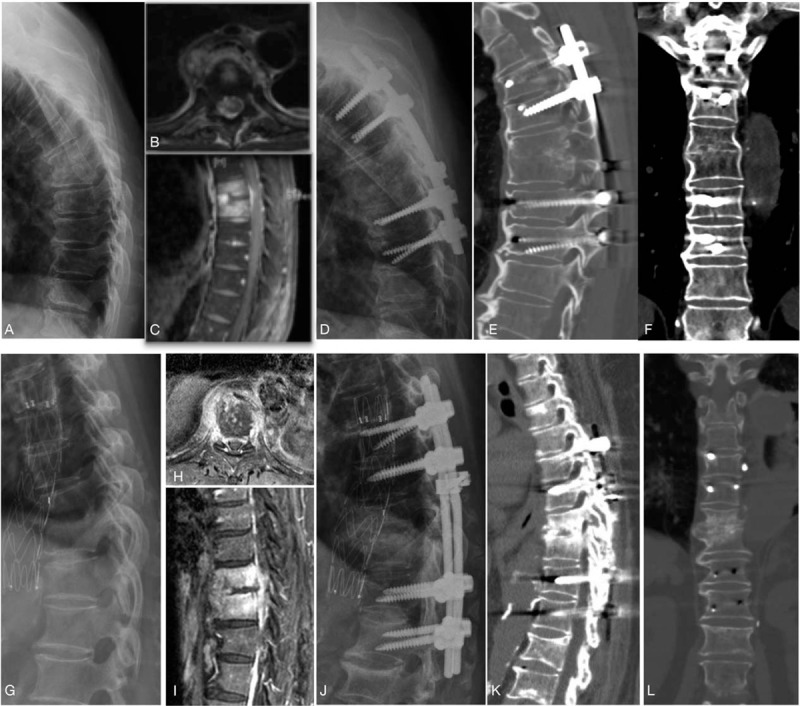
(A–F) Radiographic images of a patient treated T6–7 pyogenic spondylodiskitis with costotransversectomy. (A) Preoperative x-ray showed endplate destruction and local kyphosis at T6-7. (B, C) MRI scans revealed paraspinal abscess acumination and vertebral body signal change. (D) Costotransversectomy with posterior instrumentation T4-9 was performed. (E, F) Postoperative 6-month CT scans revealed solid fusion with continuous cortex and trabecularization across the affected segments. (G–L) Radiographic images of a patient underwent TTIDF for T9-10 pyogenic spondylodiskitis. (G) Preoperative x-ray showed severe endplate destruction and instability at T9-10. (H, I) MRI scans with contrast revealed disc destruction, vertebral body, and para-vertebral enhancement. (J) TTIDF with posterior instrumentation T7-T12 was done. (K, L) Postoperative 6-month CT scans revealed obvious bony bridge and solid fusion.
